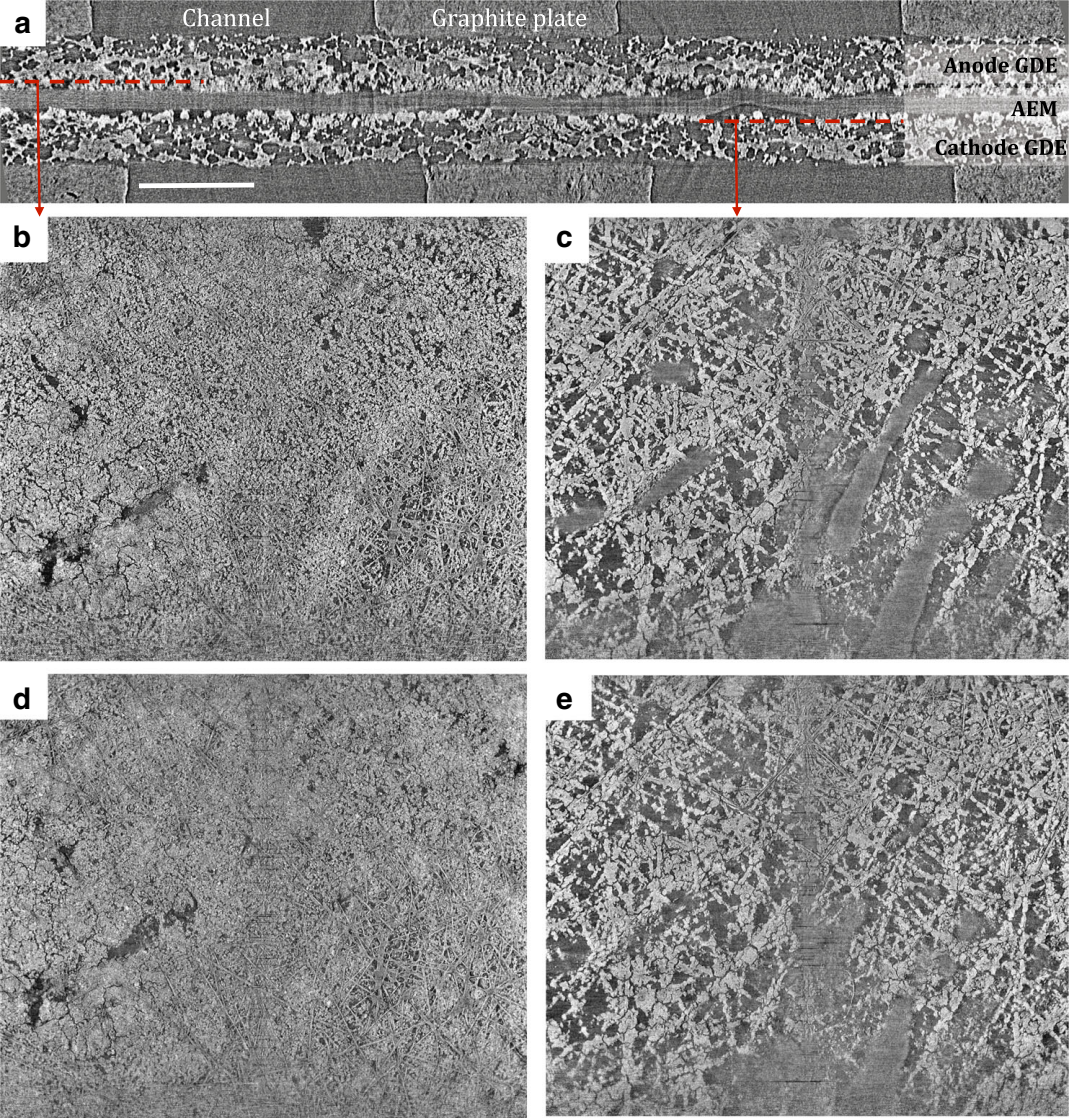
Morphology of electrodes with added PTFE. AMFC micro X-ray computed tomography images: (a) cross-section of the MEA showing the position of anode GDE, AEM, and cathode GDE of the imaged area; scale bar = 500 μm, (b), (c) longitudinal sections of anode and cathode GDEs at OCV under 100% RHs, (d), (e) longitudinal sections of anode and cathode GDEs at 0.1 V under 100% RHs. The anode and cathode used in this measurement contained 20% wt PTFE in the GDLs and 8% wt PTFE in the CLs. The anode and cathode loading were 0.70 mg_PtRu_ cm^−2^ and 0.60 mg_Pt_ cm^−2^, respectively. H_2_/O_2_ reacting gases were both fed to the cell at 200 sccm.
